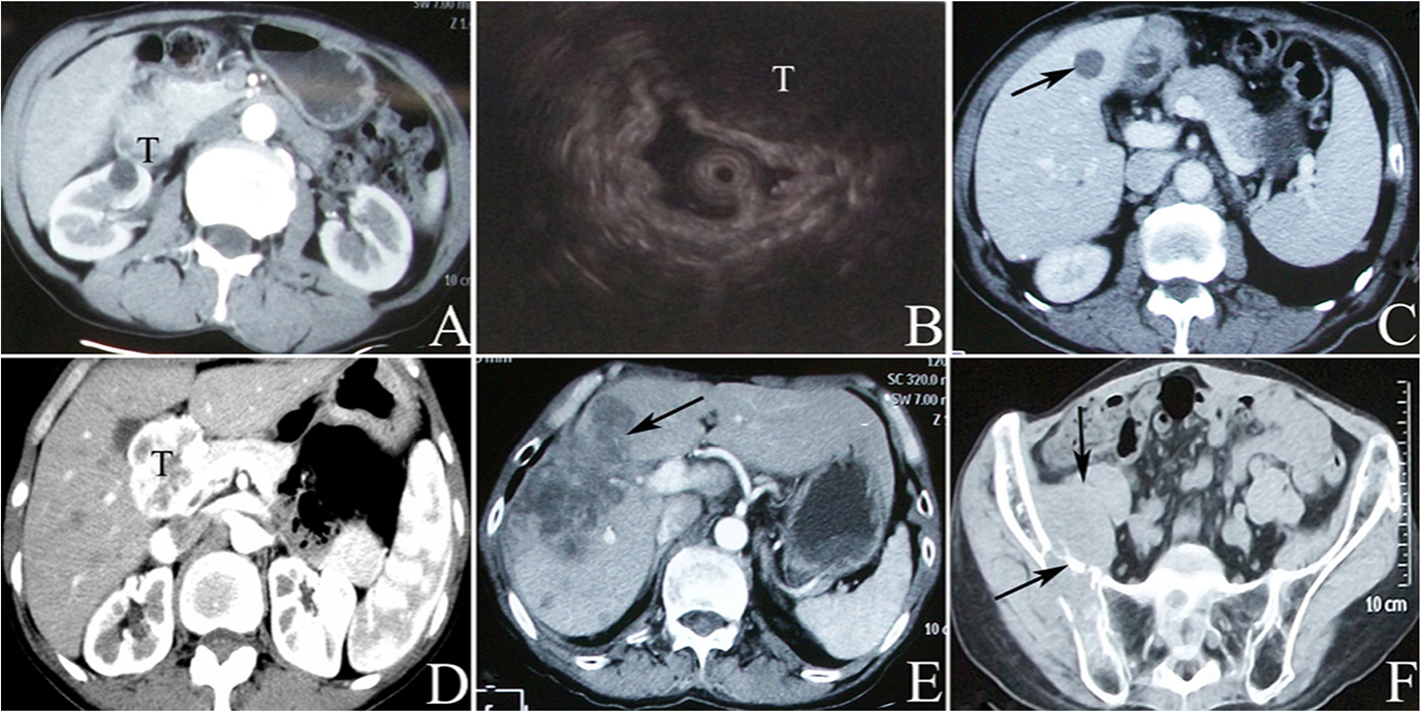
a, b: The abdominal CT scan and endoscopic ultrasonography images reveal a soft tissue mass with size of 6.5 × 4.5 cm located in the second portion of the duodenum, and with an unclear boundary with head of pancreas, right liver, and kidney. c shows a metastasis in the liver at 44 months postoperatively. d: A lump with a size of 4.5 × 3.7 cm adjacent to pancreas. e, f: Liver (mainly in the right liver lobe) and bone (multiple bone destruction of ilium) metastasis occurred 12 years after operation
